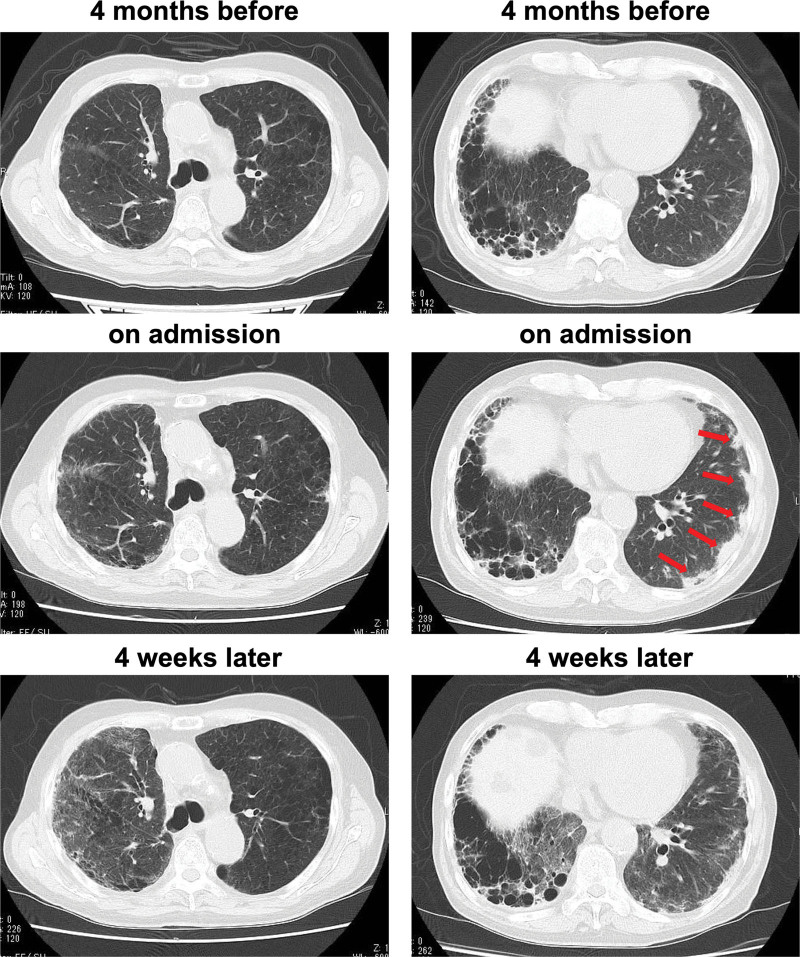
Chest computed tomography (CT) 4 months before (upper panel), on admission (middle panel) and 4 weeks later (lower panel). Chest CT revealed the multifocal pulmonary consolidations just below the pleura (red arrow) on admission, although it was not detected about 4 months before. About 4 weeks after admission (about 2 weeks after injection of prednisolone), his multifocal pulmonary consolidations were improved.
